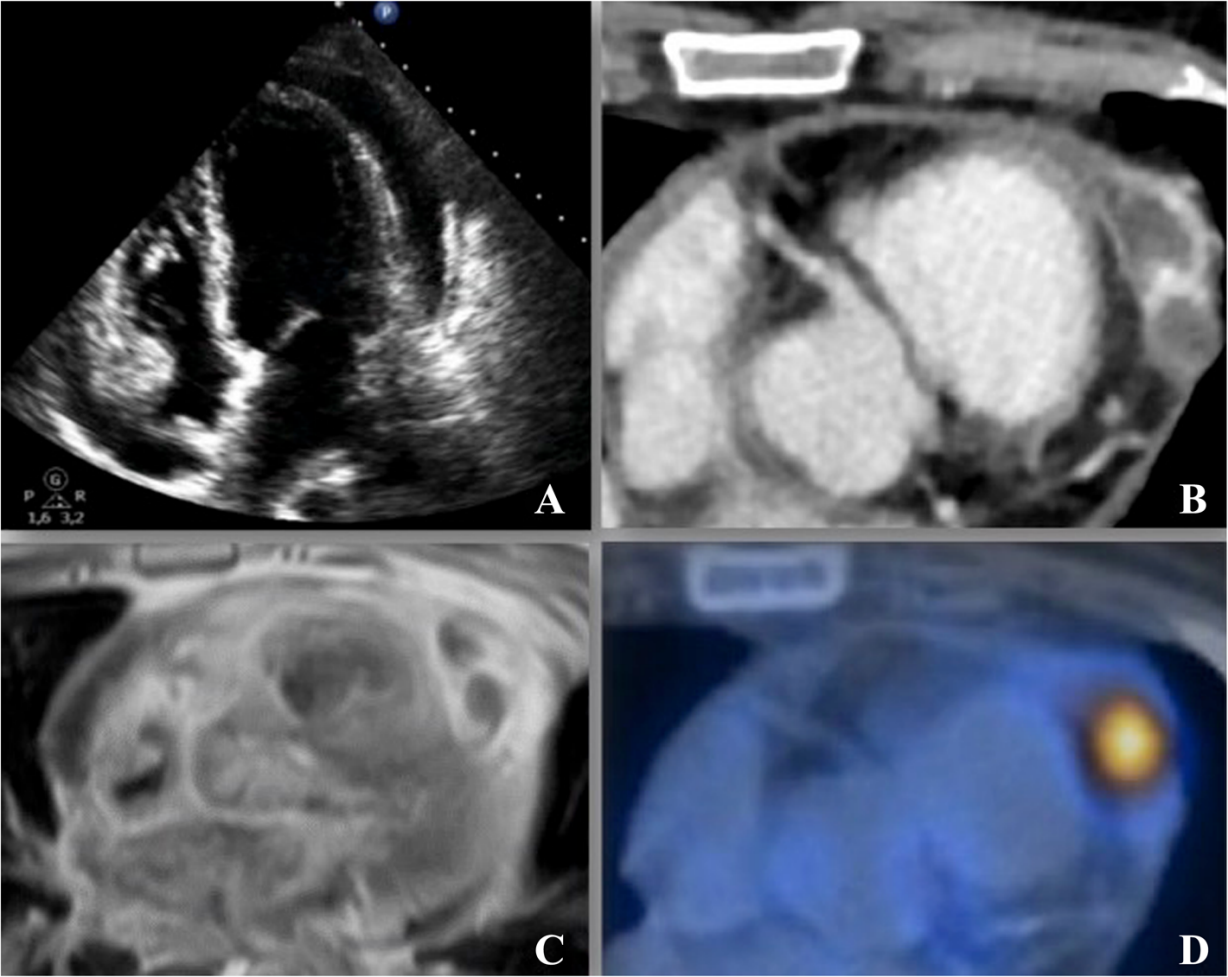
Diagnostic imaging. (A) Transthoracic echocardiograph showing ubiquitous pericardial effusion (max 22 mm) with initial signs of hemodynamic instability. (B) CT scan showing pericardial effusion localized near the right ventricle apex with marked contrast enhancement. (C) Cardiac MR showing two contiguous oval formations, on the anterior pericardial recess, (12 × 15 mm) with fluid/suprafluid content, thin walls and intense contrast impregnation. (D) ^18^F-FDG PET/CT showing a focal area of radiotracer hyperaccumulation at the anterior pericardium recess
